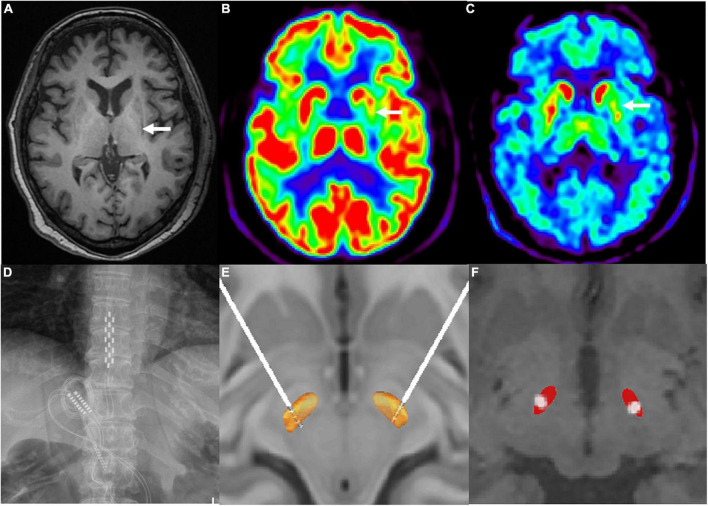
(A) Left hypointense lateral putaminal rim on T1-signal cranial magnetic resonance imaging (as indicated by the white arrow). (B)
